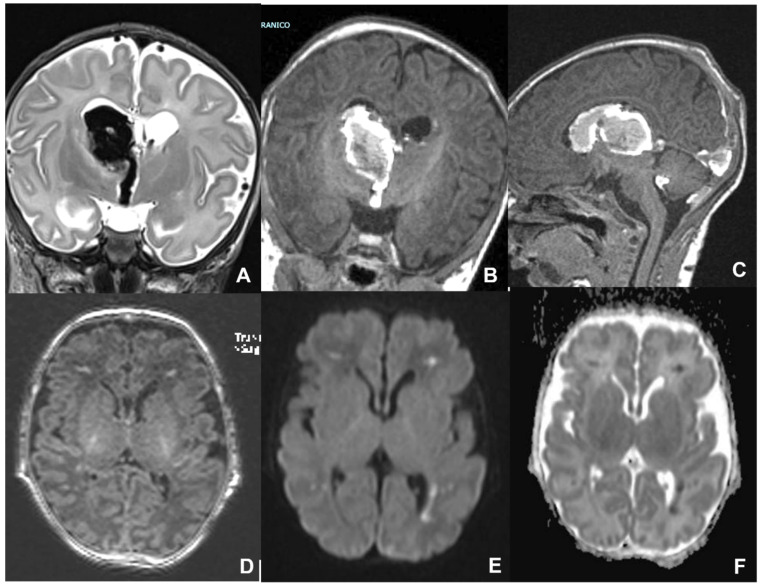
Term neonate affected by SARS-CoV2 undergoing MRI at five days after birth, showing supratentorial and infratentorial hemoventricle coexisting with subarachnoid hemorrhage on coronal T2WI-T1WI (A,B) and sagittal T1 (C). Term neonate affected by SARS-CoV-2 undergoing MRI at three days after birth, showing bilateral frontal hemorrhagic foci and linear subependymal hemorrhage on axial T1WI (D) and DWI/ADC (E,F).
